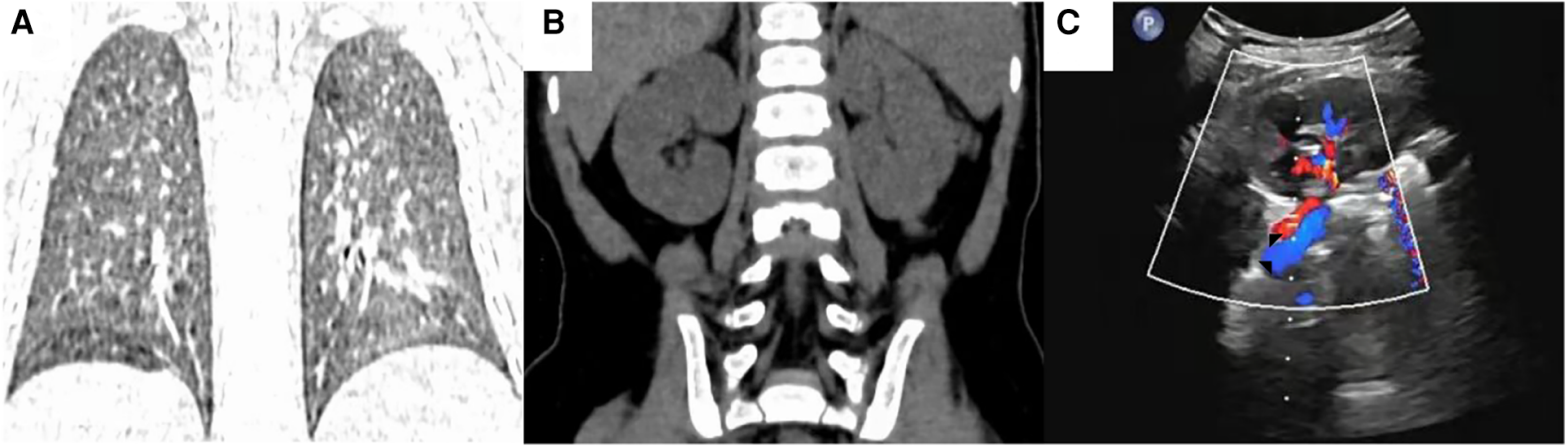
Follow up chest computed tomography (CT) imaging and renal ultrasound findings at 2 months post-surgery. (A) The chest CT coronal image showed that right diaphragm was in the normal position. (B) The chest CT coronal image showed that right kidney had returned to the abdominal cavity. (C) The renal ultrasound showed that blood flows in the right renal artery and vein were smooth. (◤: Right kidney artery, ◥: Right kidney vein).
